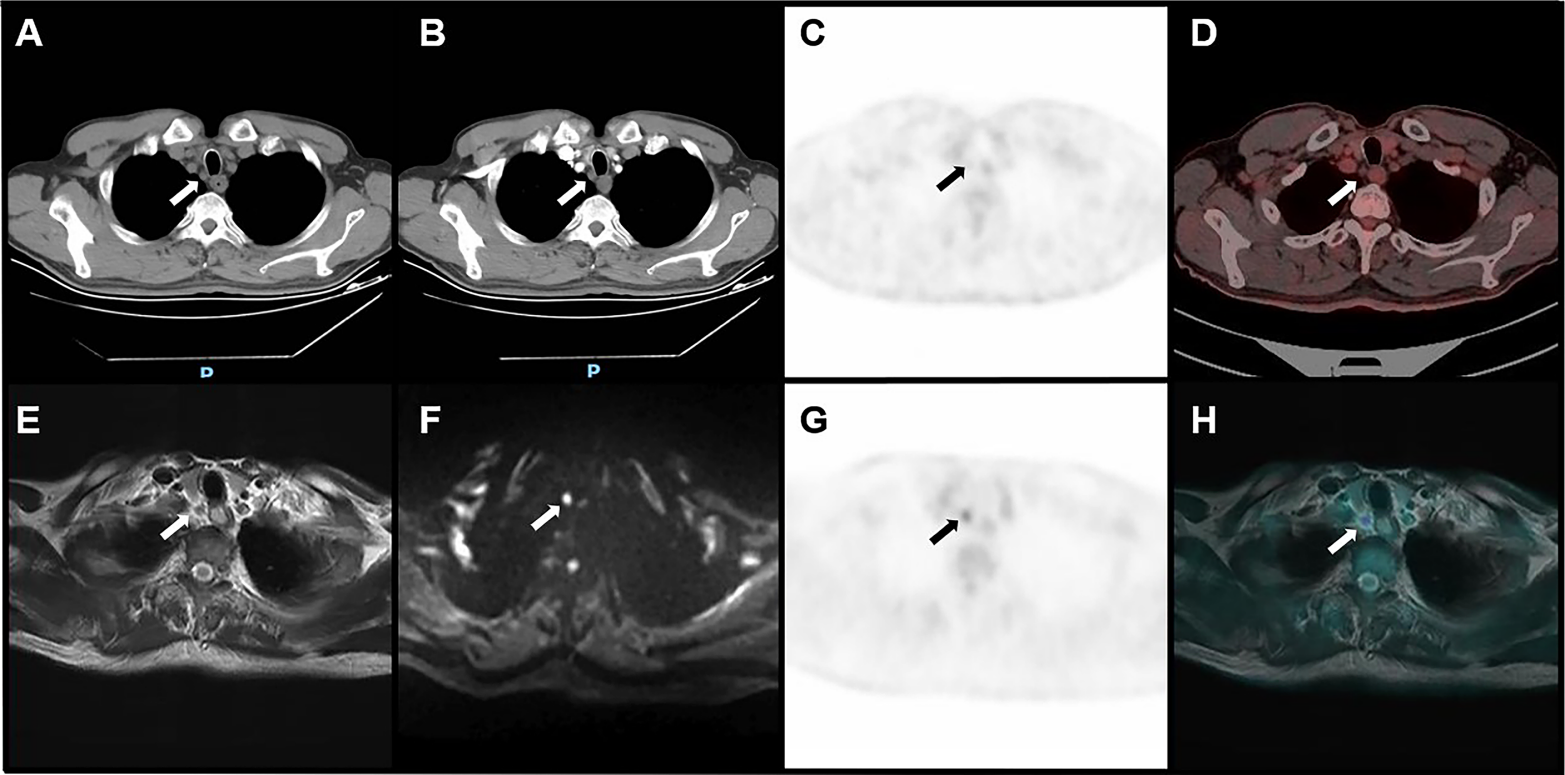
Image of a right upper paratracheal metastatic lymph node confirmed by pathology in a 66-year-old male with ESCC. CT (A), plain CT; (B), CECT showed that the short diameter of lymph nodes was 5 mm. PET/CT (C, D) showed that the uptake of the lymph node was equal to the level of the mediastinum background. Both CECT and PET/CT suggested that the lymph node was non-metastatic. PET/MRI (E–H) showed that the uptake of the lymph node was higher than the level of the mediastinum background, with slight hyperintensity on T2WI and hyperintensity on DWI, suggesting metastatic lymph nodes.
